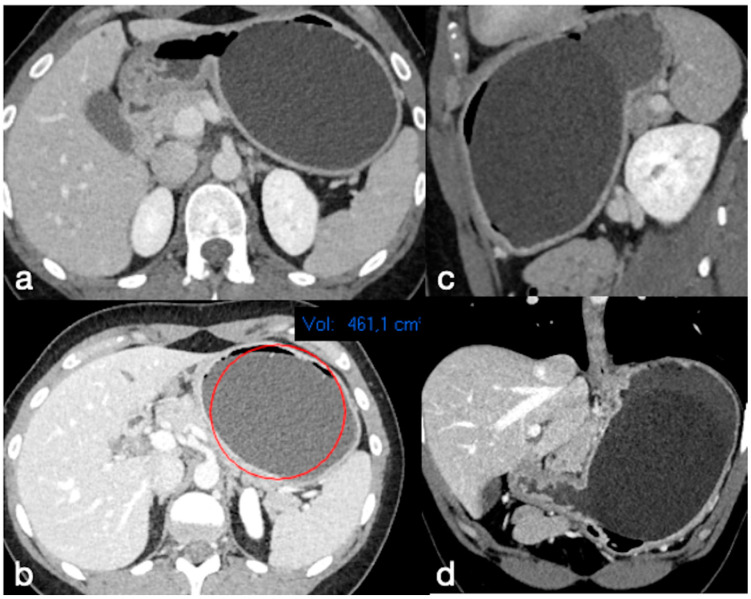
A well-placed gastric balloon in the gastric corpus and antrum seen at CT in axial (a,b), sagittal (c), and coronal (d) views, with an estimated volume of 461 cc (b).
